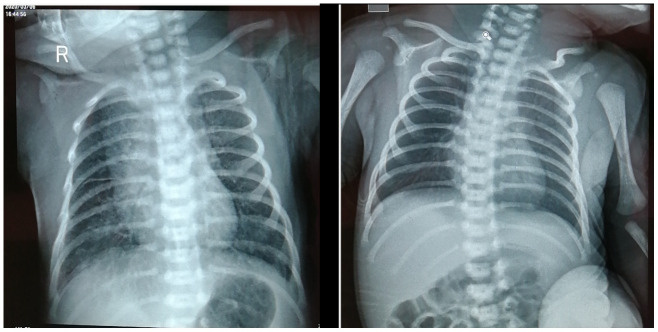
Chest X-ray of neonate on March 6^th^, 2020 (A) and March 12^th^, 2020 (B).
